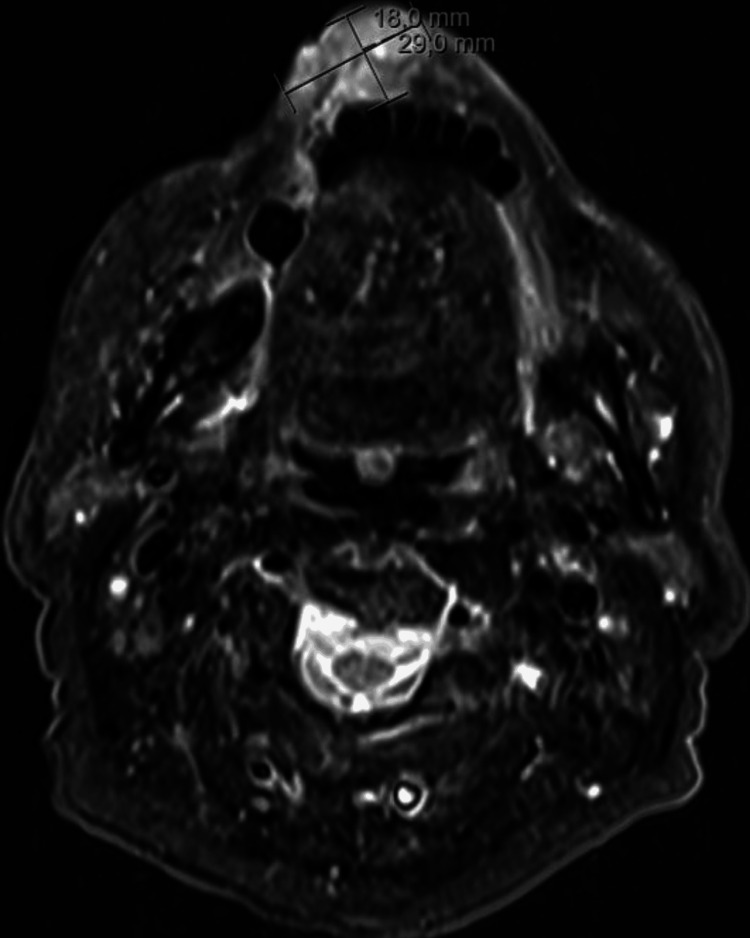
MRI scan revealing a 29 × 18 mm mass in the lower lip
